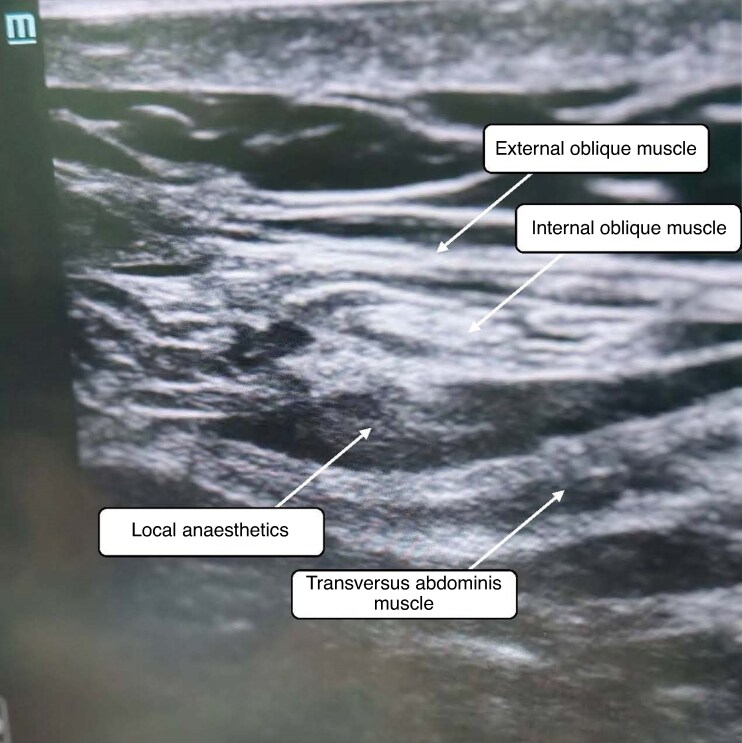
Ultrasound-guided transversus abdominis plane block
